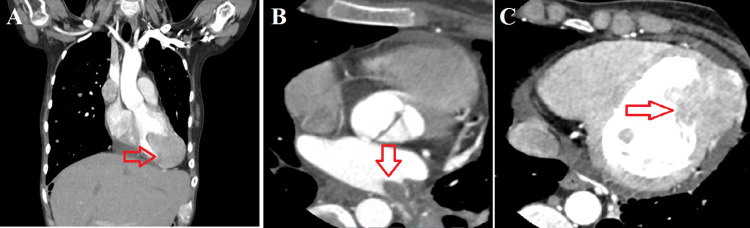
Contrast-enhanced cardiac computed tomography images of the left atrial and ventricular masses A: Coronal section view of the left ventricular mass (red arrow shows the left ventricular mass). B: Transverse section image of the left atrial mass (red arrow shows the left atrial mass). C: Transverse section image of the left ventricular mass (red arrow shows the left ventricular mass).
